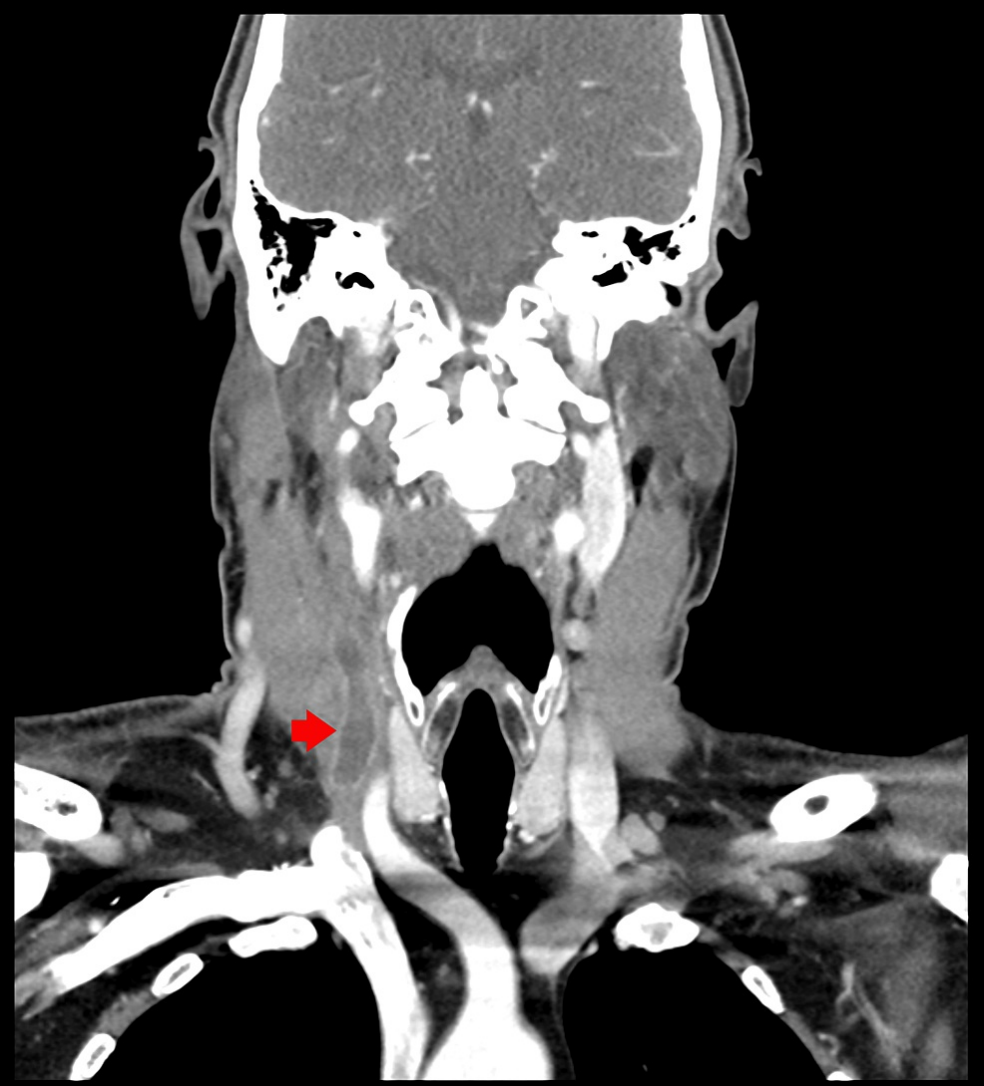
Computed tomography scan of the neck and soft tissue with contrast showing right-side internal jugular vein thrombosis (red arrow)
